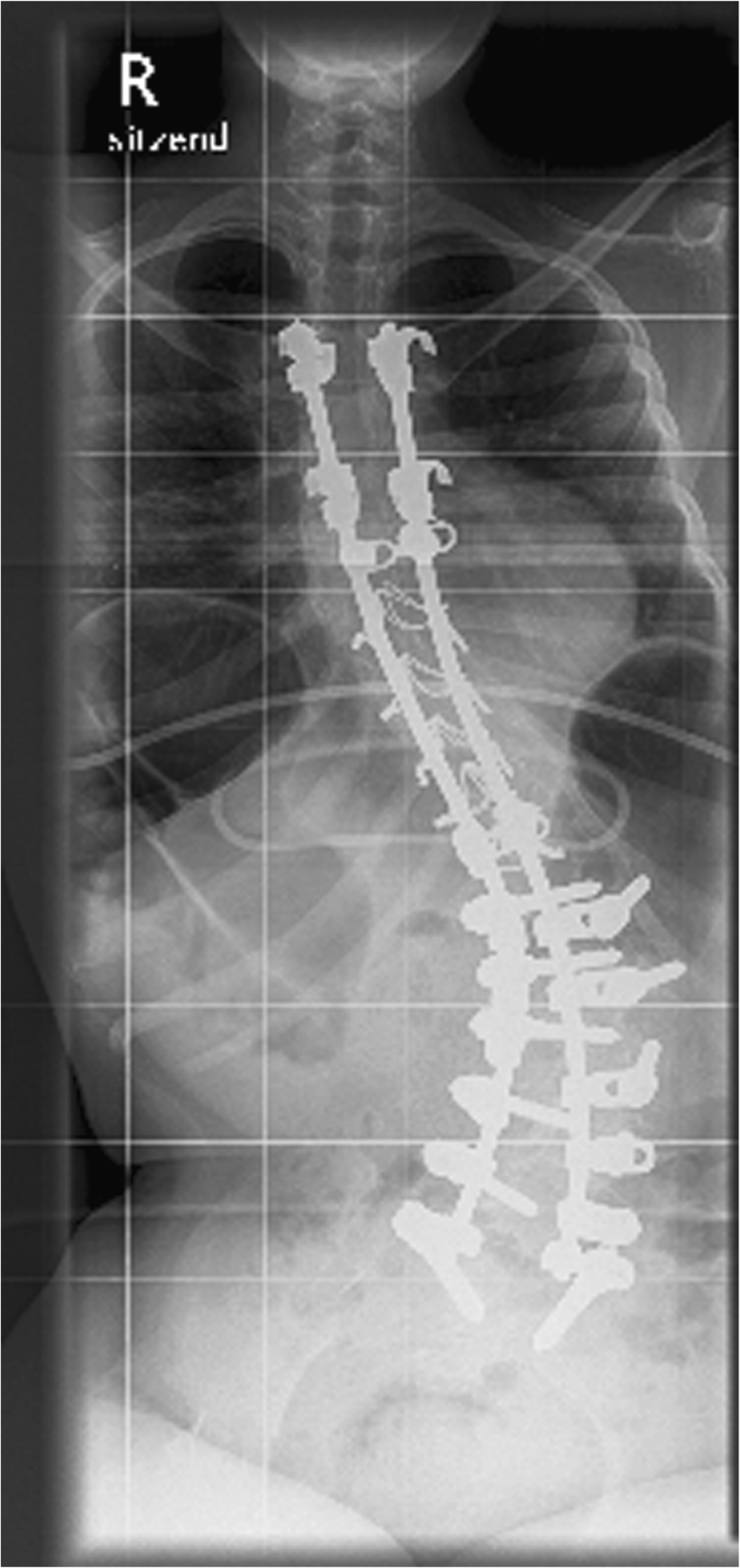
Anteroposterior X-ray of the spine at follow-up: Cobb angle of 46° (Th7-L2-L5) left-convex, 30° pelvic tilt to the left.
